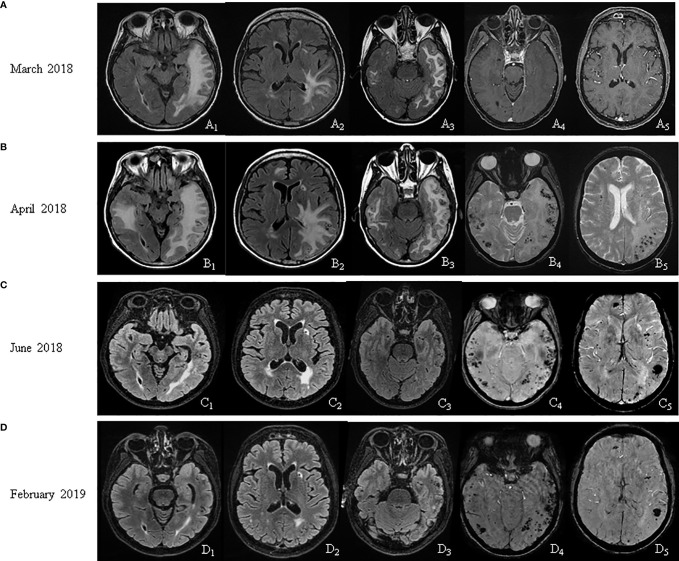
MRI follow up. (A) March 2018: FLAIR sequences showed a left temporo-parieto-occipital and a right temporal hyperintensity (A1, A2, A3); no gadolinium enhancement (A4, A5). (B) April 2018: FLAIR sequences showed an extension of the white matter (WM) hyperintensity (B1, B2, B3); gradient-echo sequences showed microbleeds (B4, B5). (C) June 2018: FLAIR sequences showed a reduction of the WM alterations (C1, C2, C3); susceptibility-weighted imaging (SWI) sequences confirmed microbleeds (C4, C5). (D) February 2019: FLAIR sequences showed a further reduction of the WM alterations (D1, D2, D3); SWI sequences corroborated microbleeds (D4, D5).
